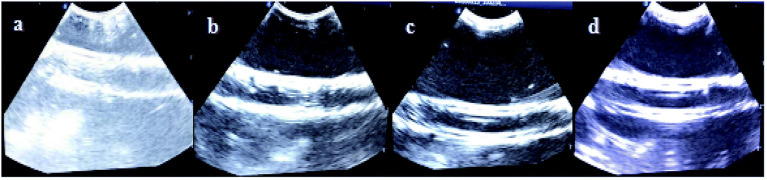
Ultrasound images of the different groups *in vitro* for: (a) normal saline,^1^ (b) span–PEG microbubbles, (c) span–PEG with CNT microbubbles, (d) span–PEG with FA–CNT–PTX microbubbles.
